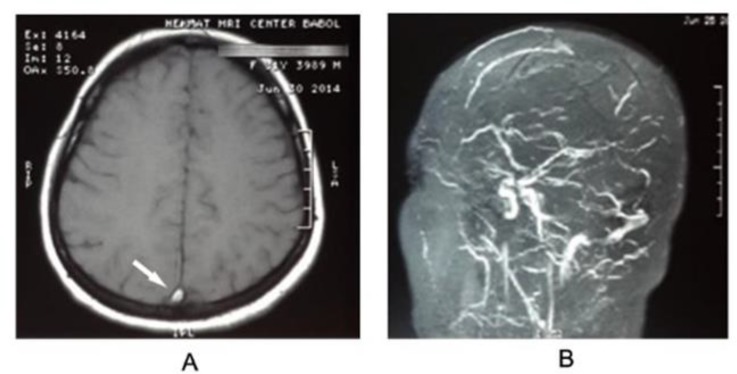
Magnetic resonance imaging of brain showed empty delta sign (A, arrowed) and magnetic resonance venography confirmed a diagnosis of cerebral venous sinus thrombosis (B
